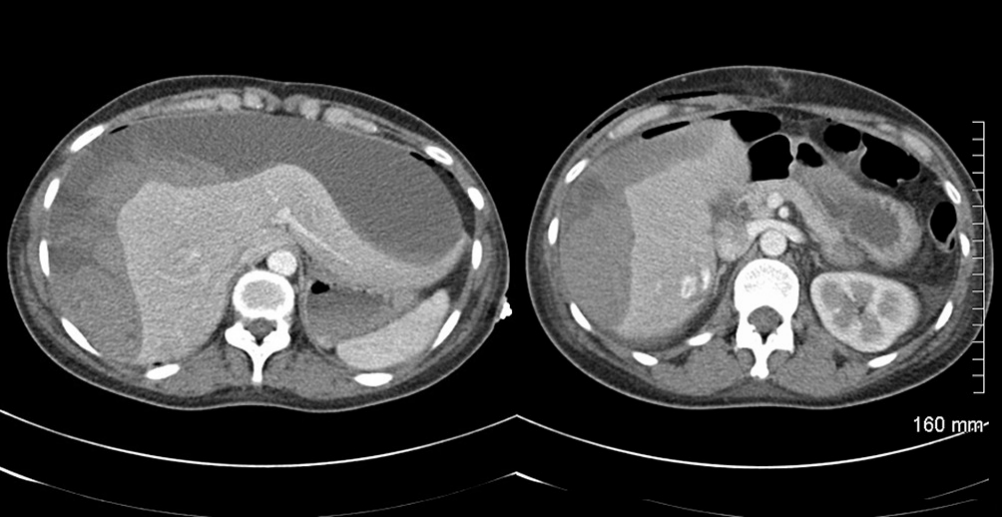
Subsequent triphasic CT identified a large subcapsular liver haematoma (left) and a hypervascular lesion on the right lobe of the liver (right).
